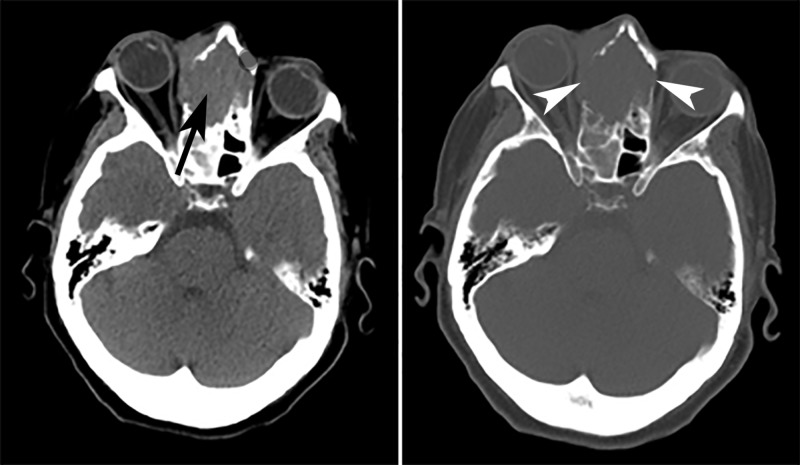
CT head Axial, soft-tissue (*left*) and bone (*right*) windowed CT images of the head demonstrate an expansile soft tissue mass (*arrow) *extending into the bilateral nasal cavity and right orbital cavity with associated osseous destructive change (*arrowheads*) of the medial orbital walls, ethmoid air cells and nasal bone. CT: computed tomography
